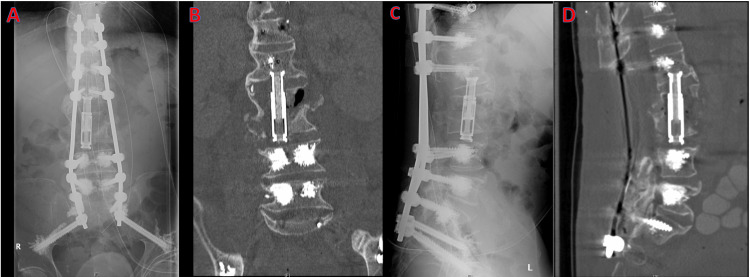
Postoperative films: (A) anteroposterior projection X-ray, (B) coronal CT scan, (C) lateral projection X-ray, and (D) sagittal CT scan. Note that the inferior surface of the graft does not contact the superior L4 endplate and the top of the graft has subsided into the L1 body.
